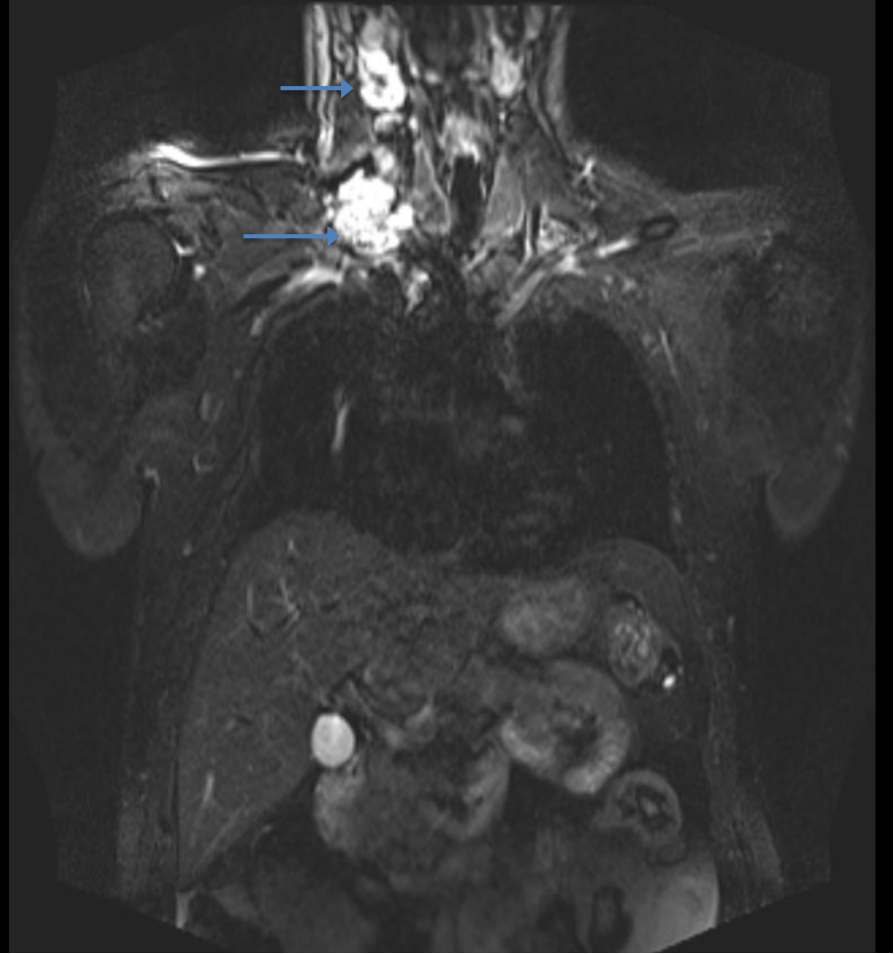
MRI of the cervical region showing the right thyroid lobe with a nodular structure and right cervical lymph nodes highly suspicious for malignancy
